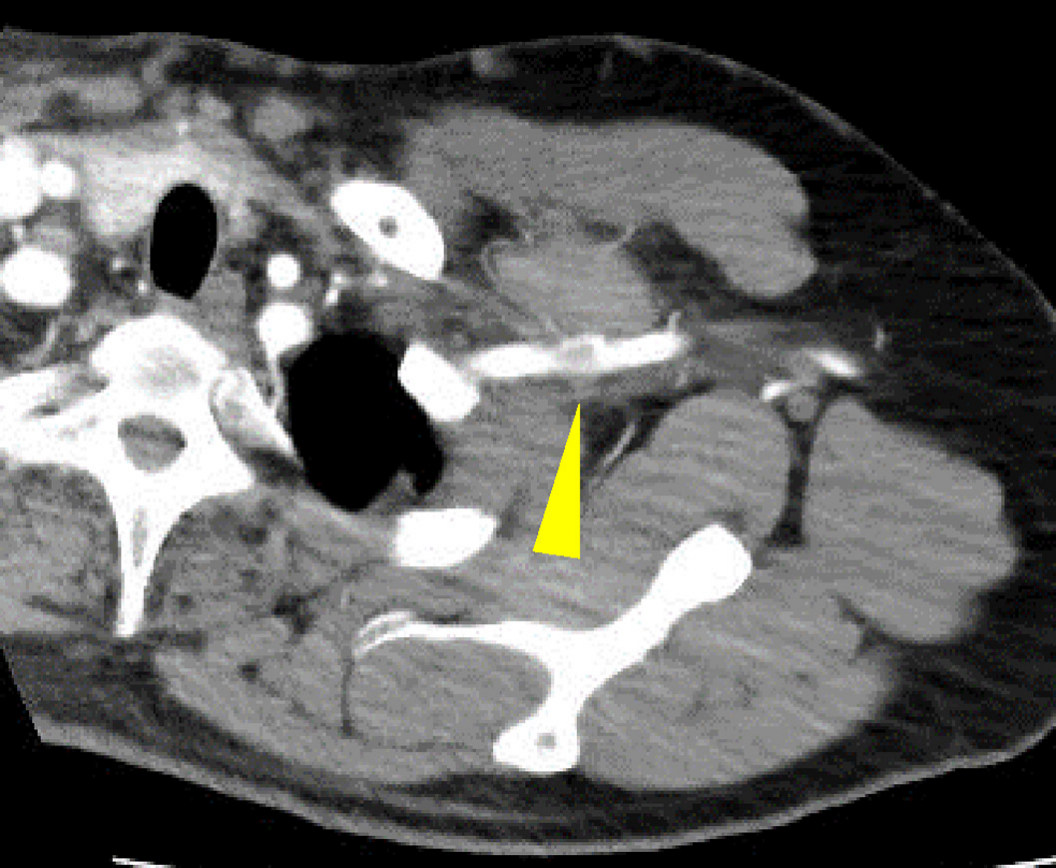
Computed tomography with contrast showed a poorly contrasted area in the superior thoracic aperture indicating Paget–Schroetter syndrome.
